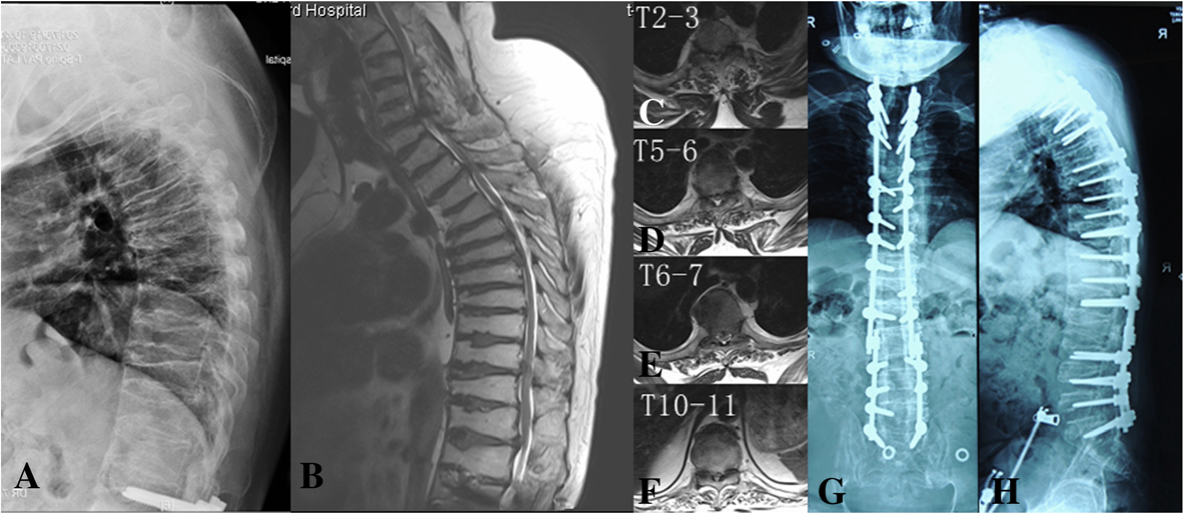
Before the second surgery, x-ray and magnetic resonance imaging of the thoracic spine showed kyphosis and multilevel canal stenosis (a–f). During the second surgery, we extensively decompressed the spinal canal from T2 to L2 and mildly corrected kyphosis of the middle thoracic spine (g, h)
